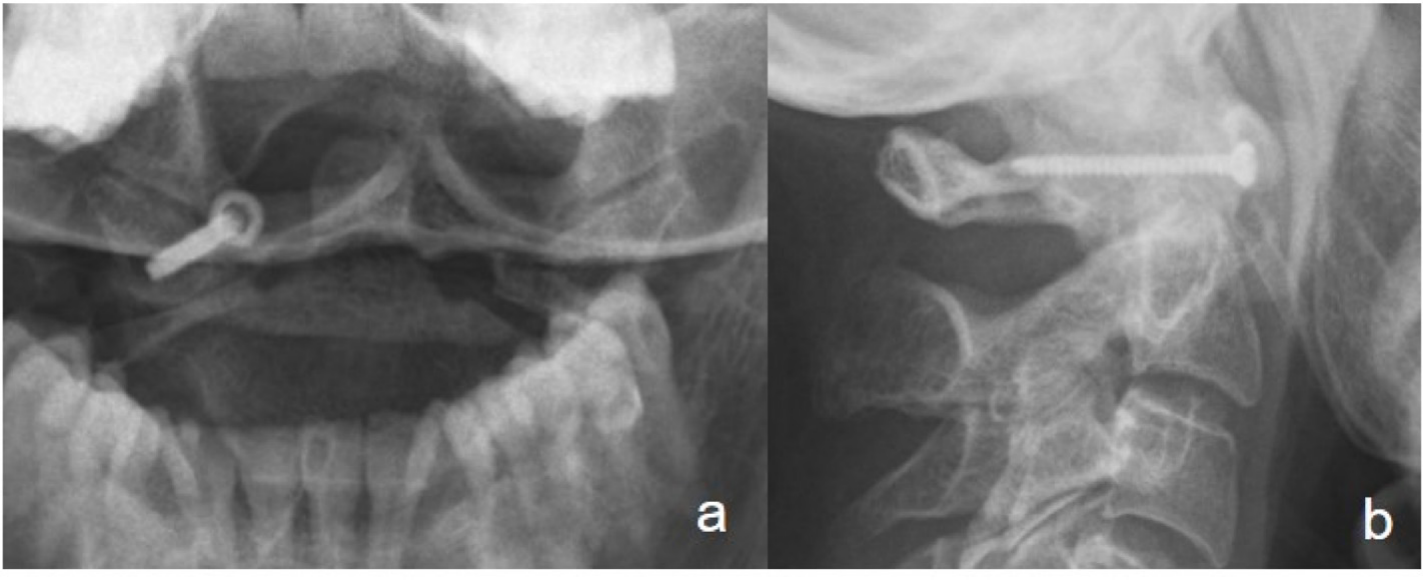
Follow-up x-ray (a/b) after 6 months with the unchanged position.
